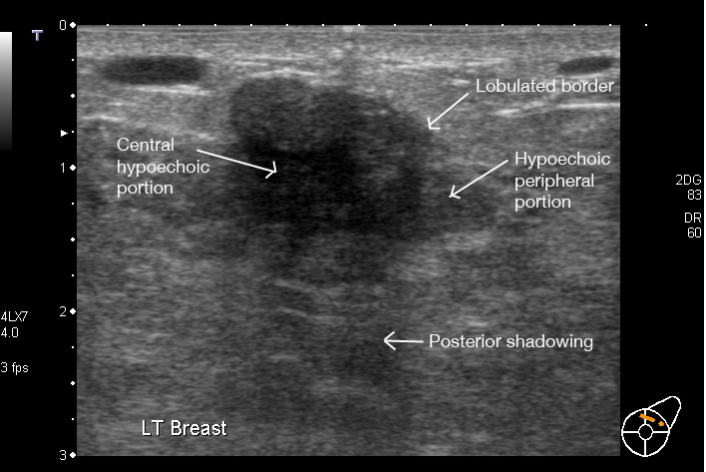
Fibroadenoma in a lactating breast – incorrect. This fibroadenoma was diagnosed and investigated prior to pregnancy. It appears ultrasonically as a heterogeneous lobulated mass with reduced sound transmission.
